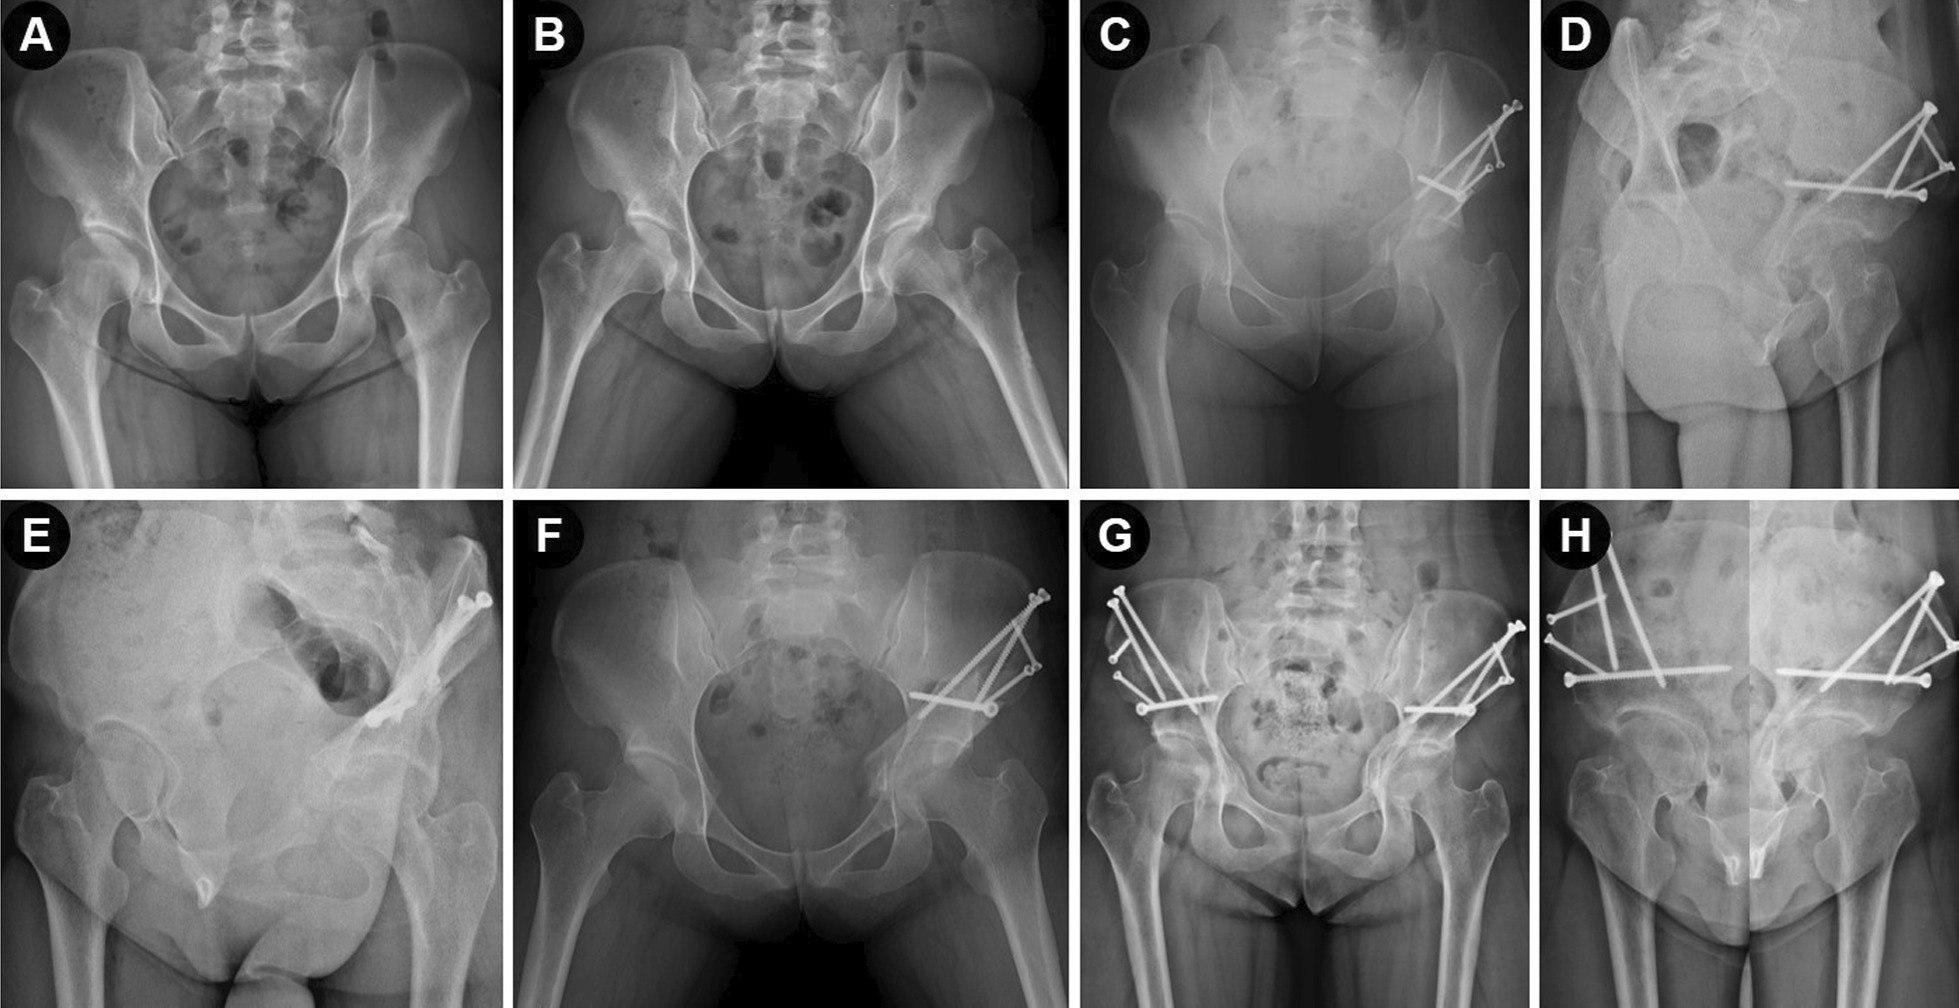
Preoperative radiograph and post-operative correction of a 22 years old woman with bilateral hip dysplasia. She was treated by Bernese PAO using the double-incision approach. A, B Preoperative radiographs of pelvis, showing bilateral acetabular dysplasia. C, D Postoperative anteroposterior pelvic radiograph and false-profile radiograph of the left hip at 2 months. E, F Preoperative radiographs of the right hip. G, H Postoperative anteroposterior pelvic radiograph and false-profile radiograph at 1 year (left hip) and 6 months (right hip)
